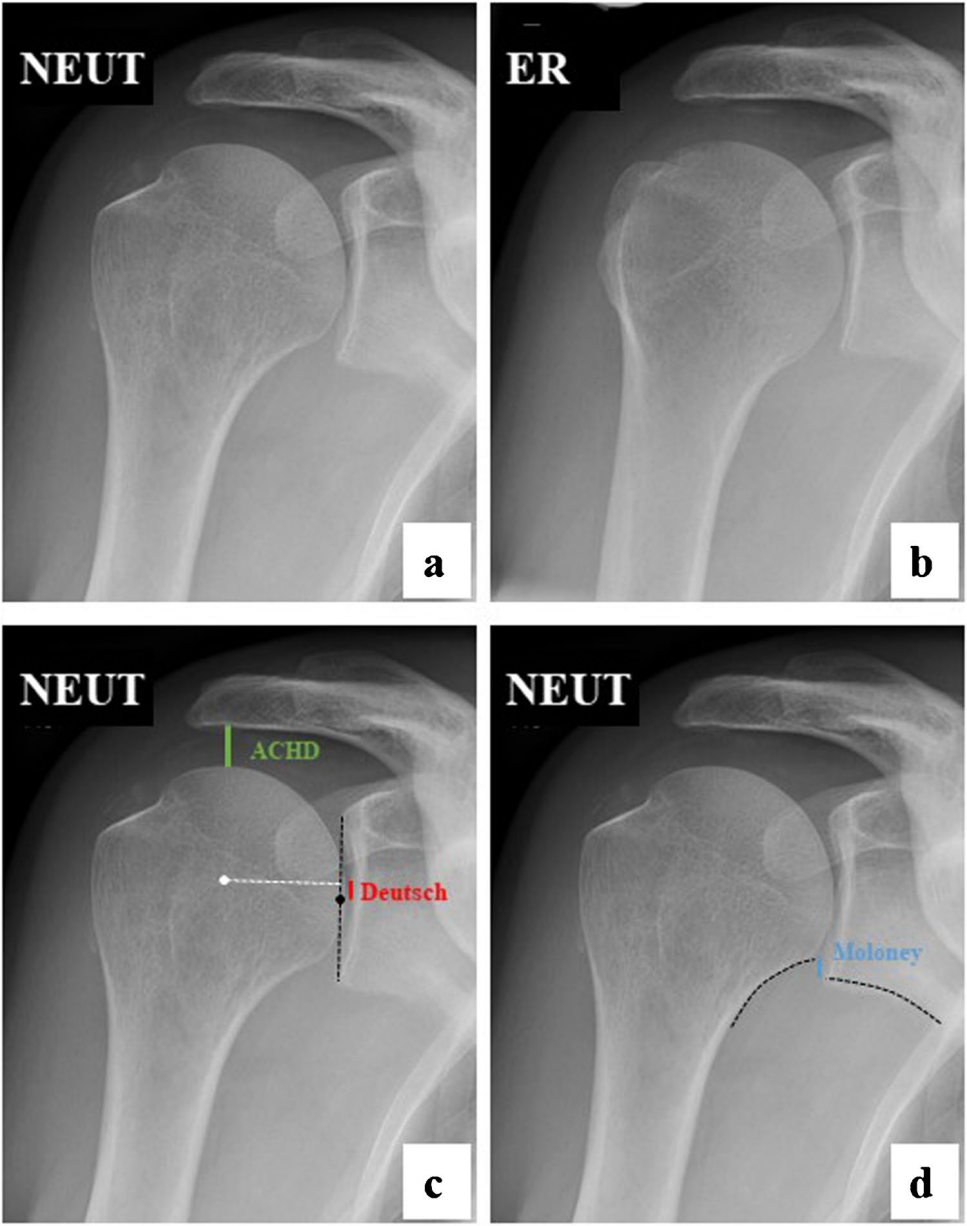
Oblique anteroposterior radiographs of a right shoulder in neutral position (NEUT) and in external rotation (ER). a, b The rotation of the humerus alters the projected shapes of the humeral head and the calcar, influencing the measurements for glenohumeral centering. c Glenohumeral centering was quantified by the acromiohumeral distance (ACHD), by the distance of the center of the humeral head to the center of the glenoid in cranio-caudal direction according to Deutsch, and d by the congruity of the scapulohumeral arch, known as the Moloney line
